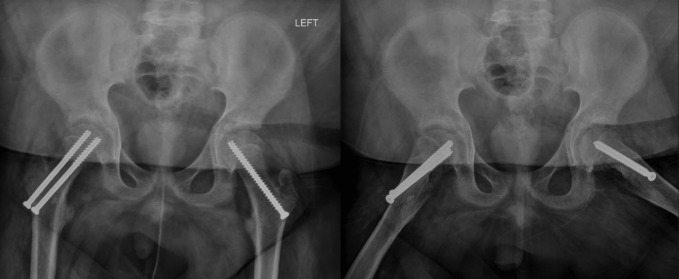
Postop radiographs (AP pelvis and frog-leg lateral).
